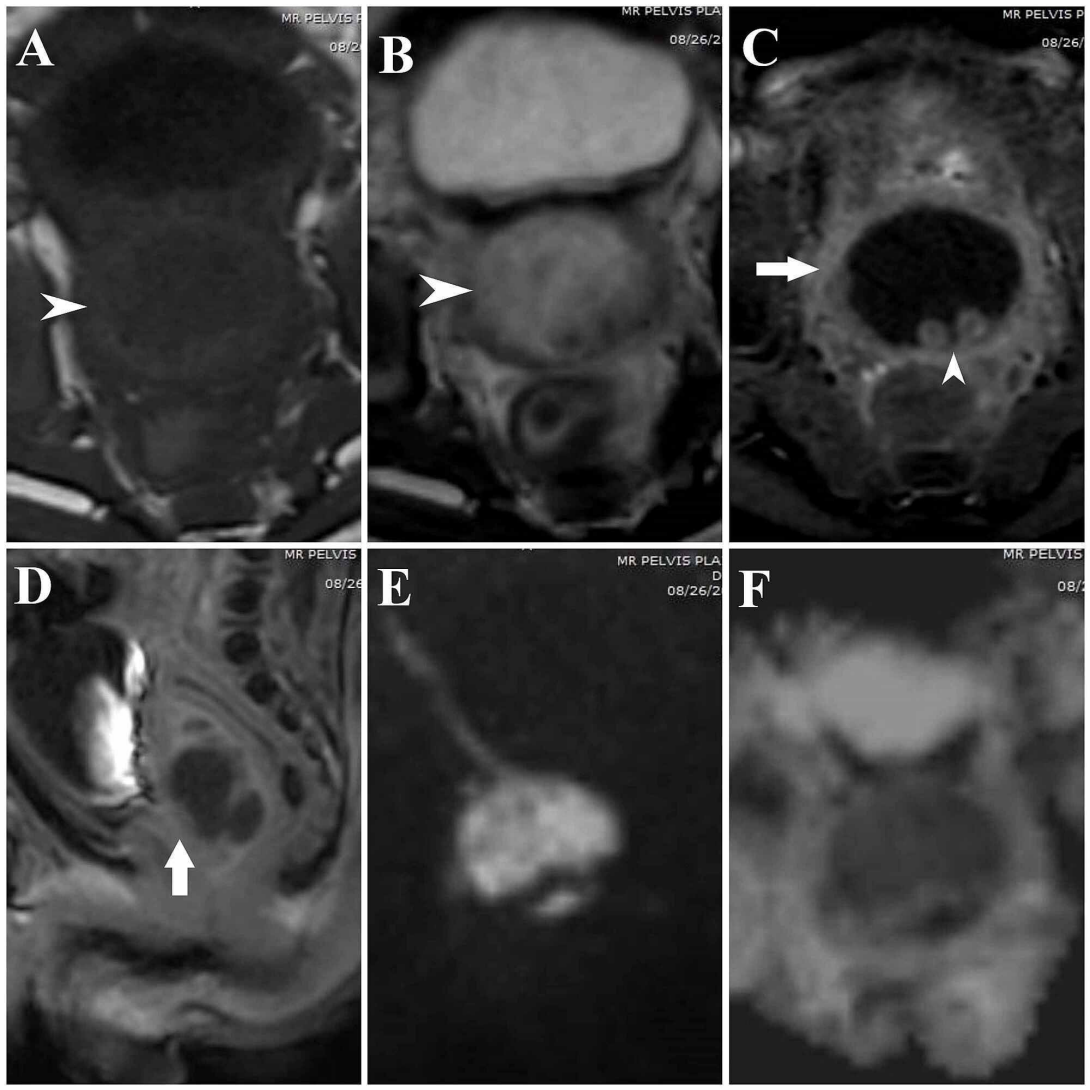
MRI pelvis plain and contrast images A – Axial T1-weighted image, arrowhead shows significantly enlarged and hypointense prostate gland. B – Axial T2-weighted image, arrowhead shows significantly enlarged and hyperintense prostate gland. C – Axial T1-weighted post-contrast image, horizontal arrow shows significant peripheral contrast enhancement of the prostate gland, and the vertical arrowhead shows peripherally enhancing ejaculatory ducts. D – Sagittal T1-weighted post-contrast image shows residual contrast in the urinary bladder. The vertical arrow shows significant peripheral contrast enhancement of the prostate gland. E – Diffusion-weighted image shows hyperintense enlarged prostate gland. F – Apparent diffusion coefficient (ADC) mapping corresponding to image E shows hypointense prostatic parenchyma with a low ADC signal suggestive of restricted diffusion.
